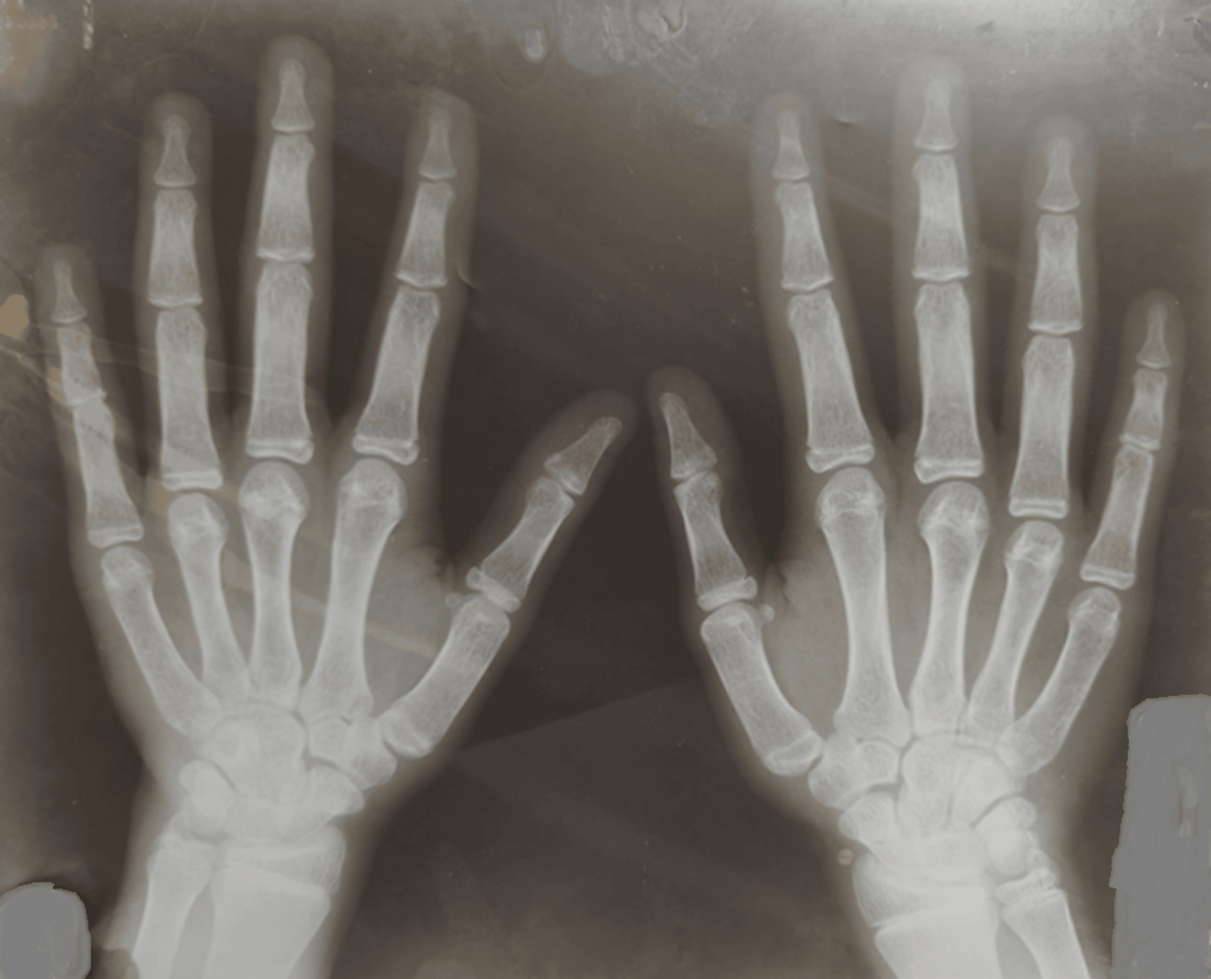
X-ray of both wrists and hands (posteroanterior view) at a chronological age of 10 years showing a bone age of 17 years.
